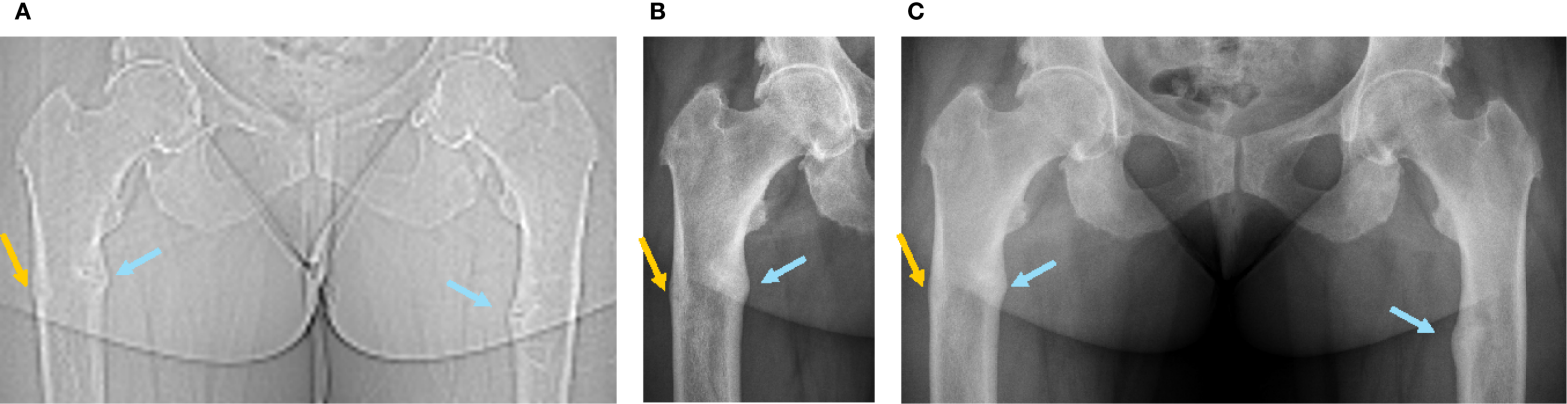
Radiographic images of the femoral fractures before and after introduction of burosumab treatment (first burosumab injection: 04.01.2021). (A) SPECT-CT topogram before introduction of burosumab treatment (16.09.2020). (B) Five weeks after first burosumab injection (12.02.2021). (C) Three and a half months after first burosumab injection (23.04.2021).
